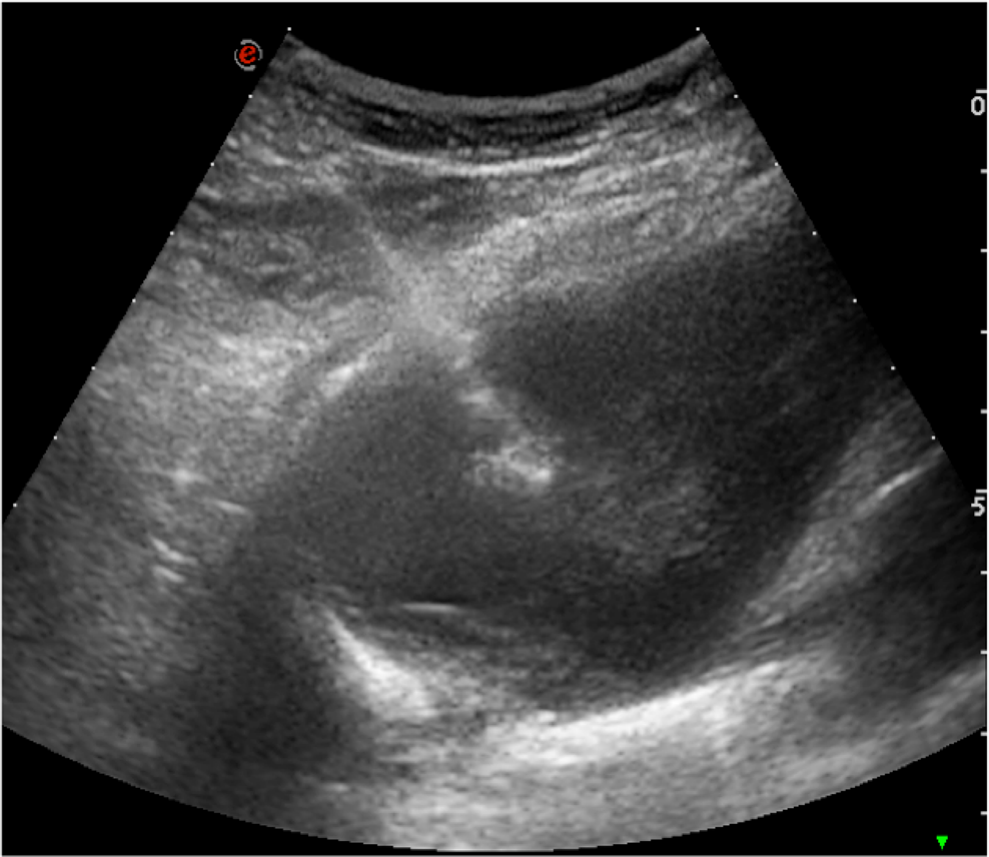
Ultrasound-guided chest biopsy of a pulmonary nodule in the right lower lobe
